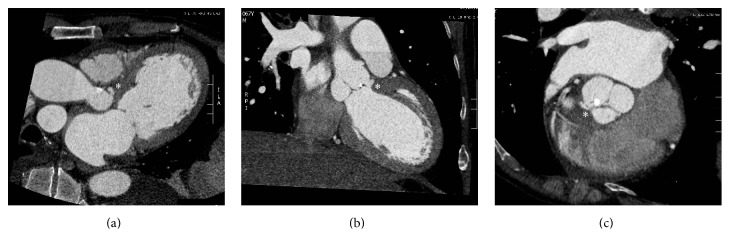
Multiplanar, reformatted oblique views of cardiac CTA (a, b, c) demonstrating the small pseudoaneurysm (*∗*) involving the aortic annulus and the mitral-aortic intervalvular fibrosa.
